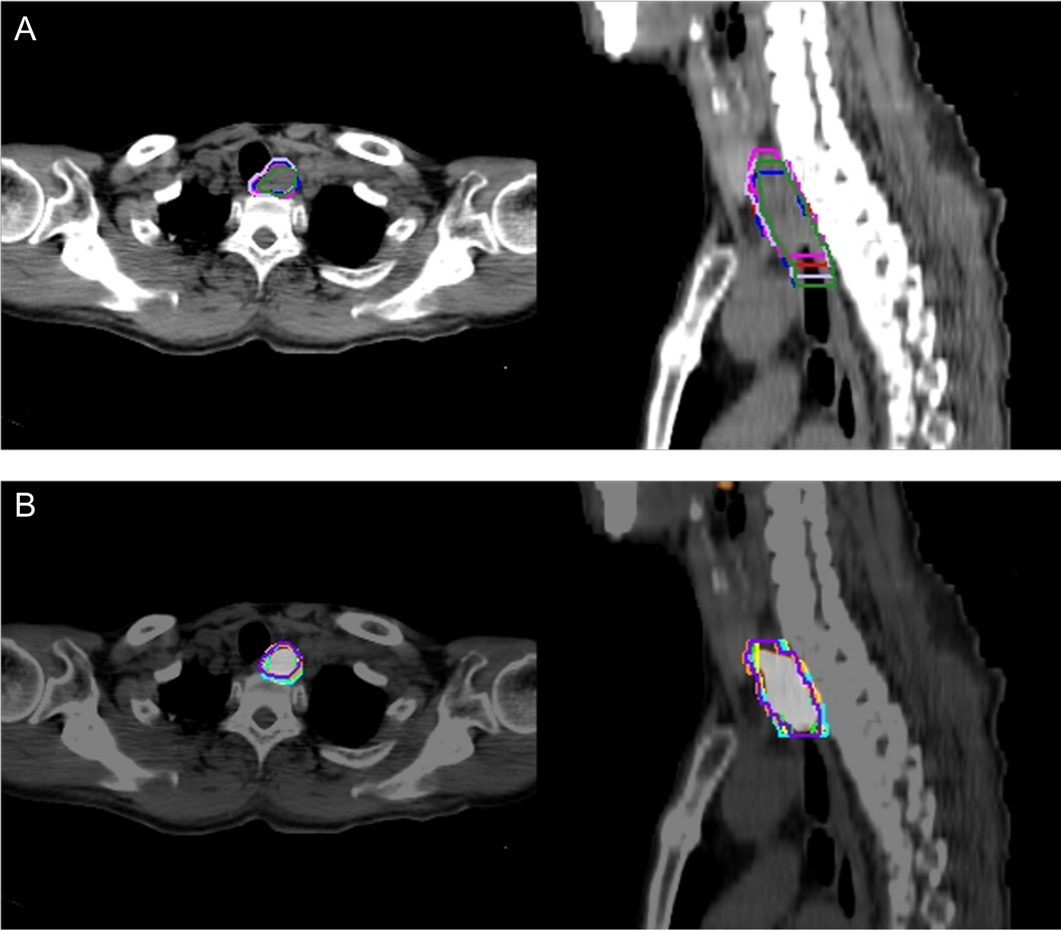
Determining the gross tumor volume (GTV) in a patient with cervical esophageal cancer (cT3N0M0). Each panel includes an axial (left) and a sagittal (right) image. (A) GTVs defined by five observers based on computed tomography (CT)-alone images. The conformality index (CI) was 0.41. (B) The CI improved to 0.59 with positron emission tomography (PET)/CT images.
